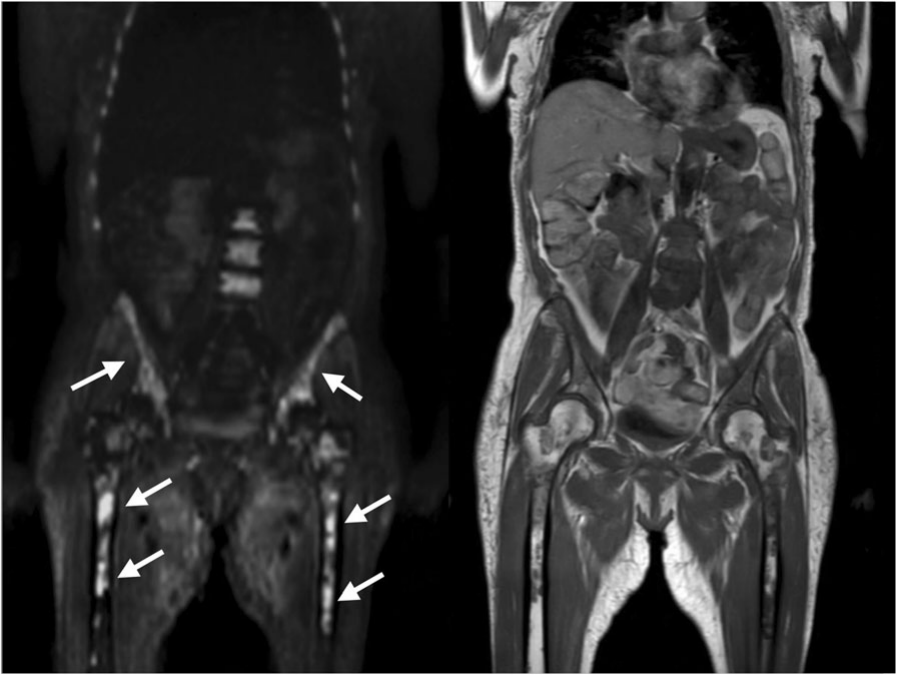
Representative coronal slice of a WB-T1w (left) and WB-DWI (right) of the same MM patient. MM focal lesions visible on left femoral head, right iliac wing and lumbar vertebral bodies, show hyperintense signal on b800/1000 image (right) and hypointense signal on T1w image (left)
